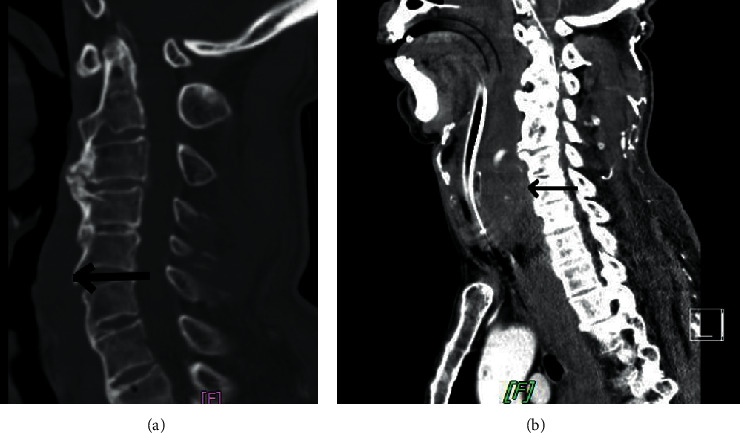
The dynamic changes from an initial computed tomographic scan of the cervical spine (a) to a later computed tomographic scan of the neck (b). Retropharyngeal hematoma (arrow) expanded significantly 4 hours after injury. In Figure 7(b), the patient had undergone tracheal intubation, and extravasation of contrast media in the hematoma indicated an active bleeder.
